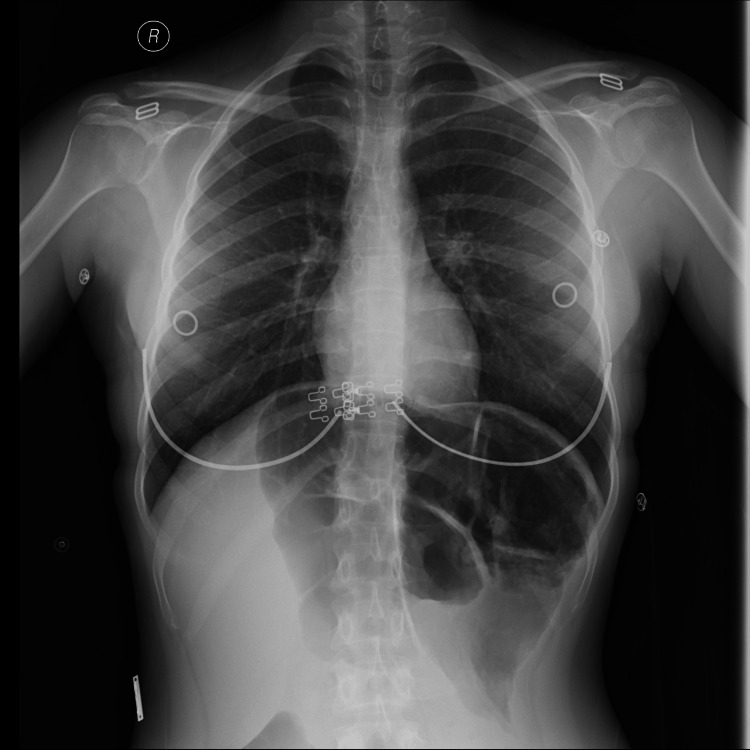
Upright chest x-ray suggesting dilated distal colon without pneumoperitoneum
